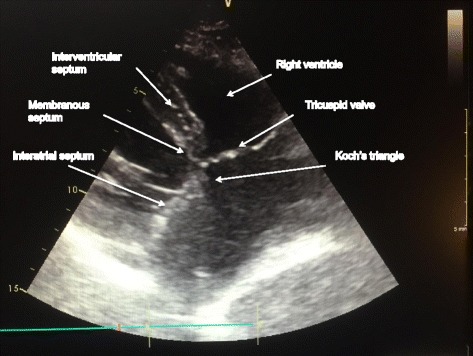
Transthoracic echocardiography demonstrating the absence of recurrence in the right atrium close to the Koch’s triangle
